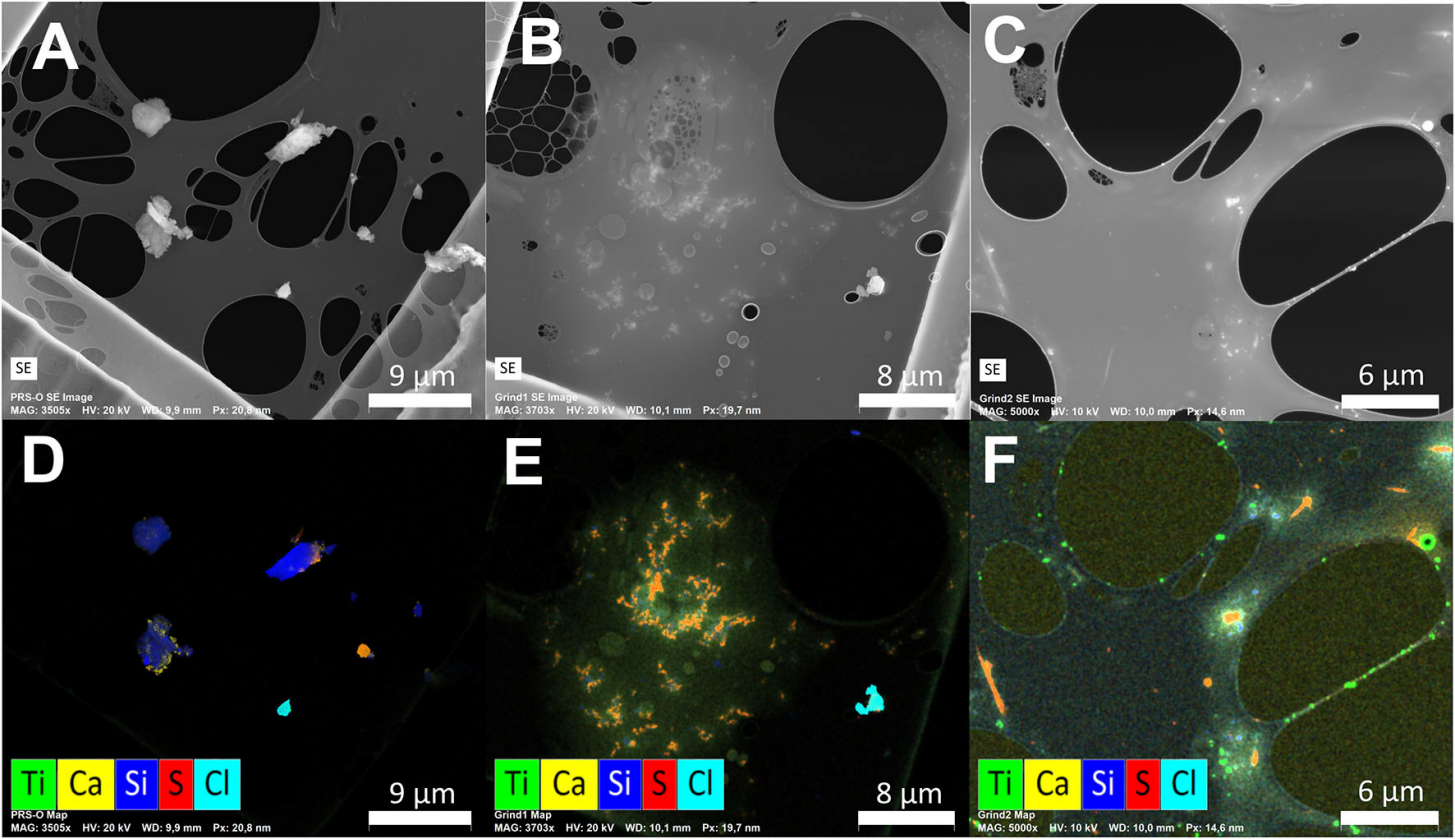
Representative SE images (A–C) and overlaying EDS maps of select elements (D–F) from the PRS-O sample (A,D), Grind1 sample (B,E), and Grind2 sample (C,F). The EDS maps were generated based on X-ray counts in the energy range corresponding to an emission from the given element. The individual element maps were those used for segmentation prior to quantification.
